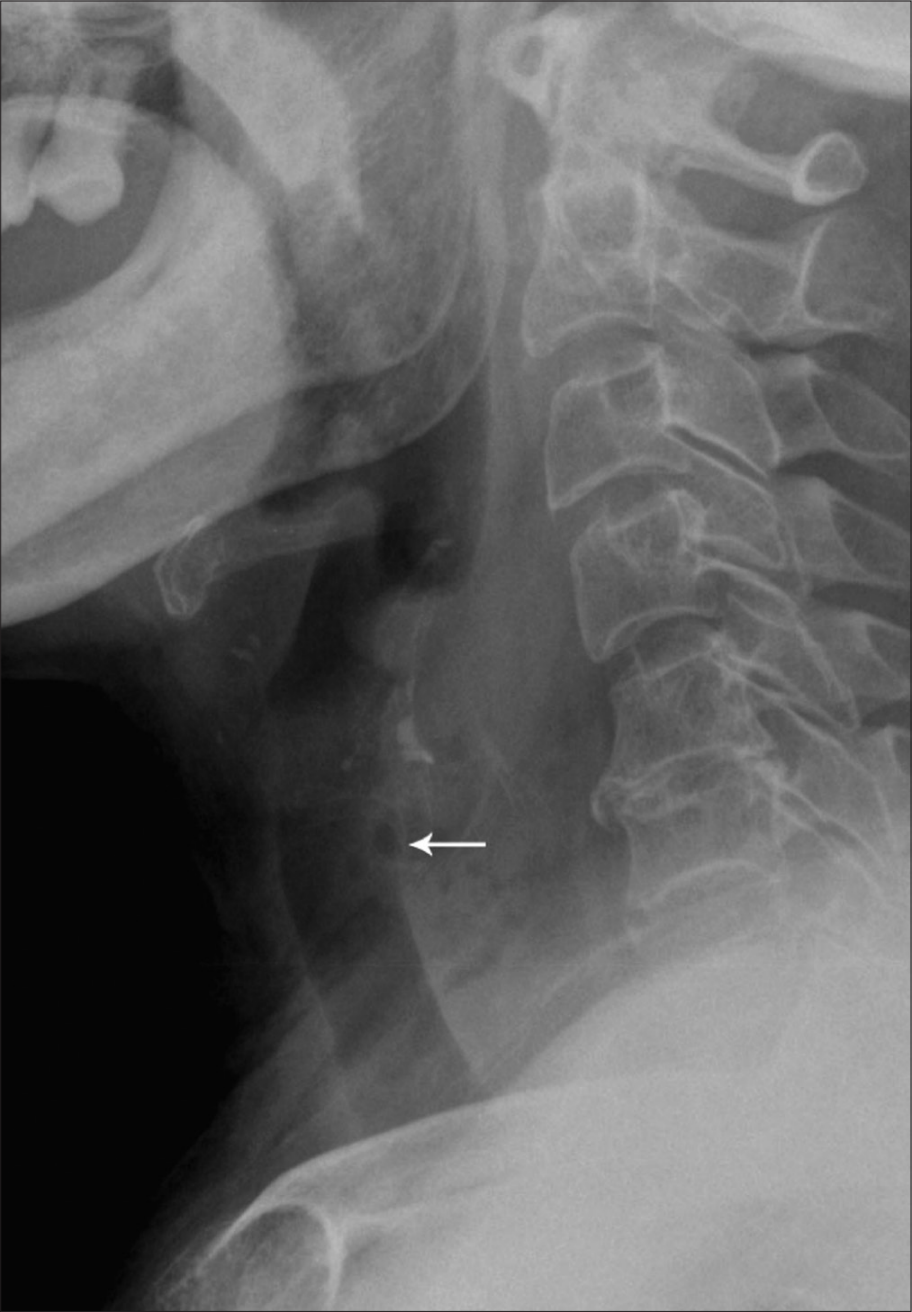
Lateral radiograph of a patient shows widening of the prevertebral space, with small air locules within (arrow), findings consistent with a retropharyngeal abscess. A noncontrast CT scan (not shown) revealed the presence of an underlying FB, which is obscured on the radiograph by the surrounding soft tissue
